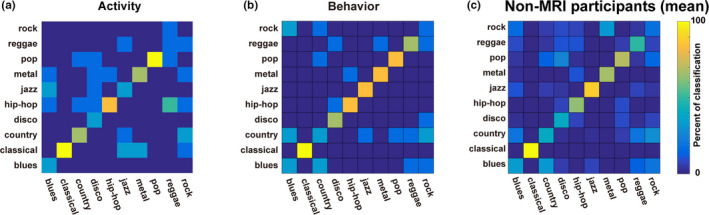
The modulation‐transfer function (MTF) model explains the genre representational specificities of brain activity and behavior.(A)The confusion matrix based on the brain activity of participant ID01 using the decoding model approach. For each column of correct music genres, the percentage of classified music clips was plotted on the row of classified music genres.(B)The confusion matrix based on the behavioral data of participant ID01.(C) The mean confusion matrix based on the behavioral data of the non‐MRI participants. For each column of music genres, the percentage of correctly classified music clips was plotted on the row of the classified music genres. Error bar, SD.
